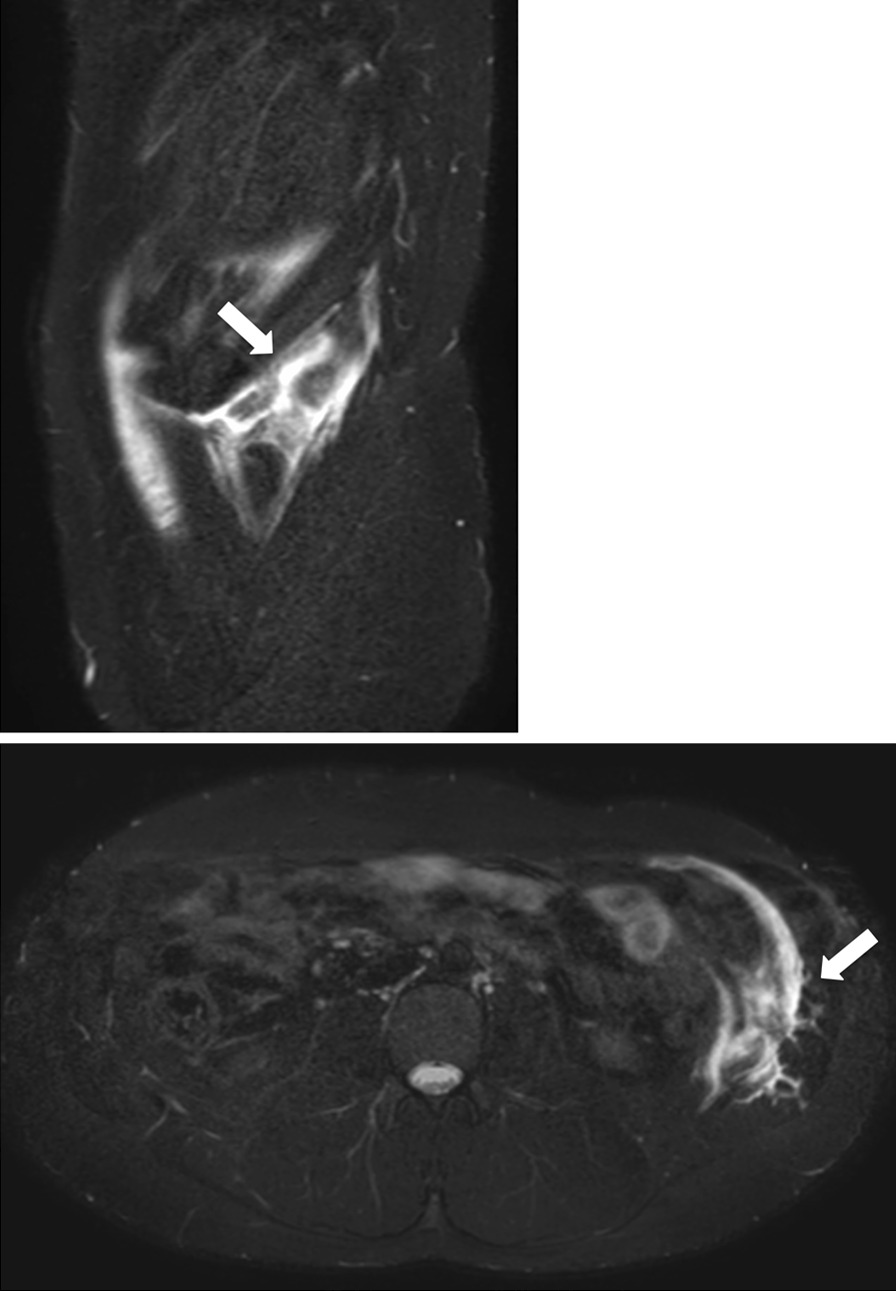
Sagittal and Axial MRI of internal oblique muscle strain. On sagittal and axial MRI with fat suppression, an area of high signal intensity is visible in the lower rib area (white arrow)
